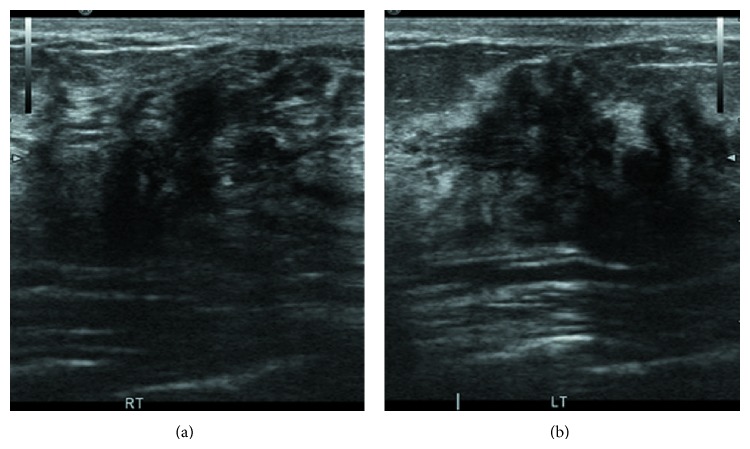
The ultrasound examination demonstrated a solid hypoechoic mass with an irregular shape and a lobulated margin both in the upper outer quadrant of the right breast (a) and below the nipple of the left breast (b).
